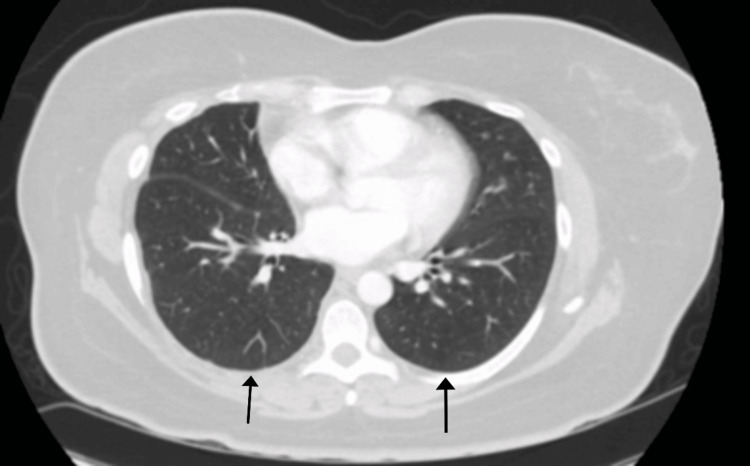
CT chest showing complete resolution of pleural effusion after 5 days of ceftriaxone
